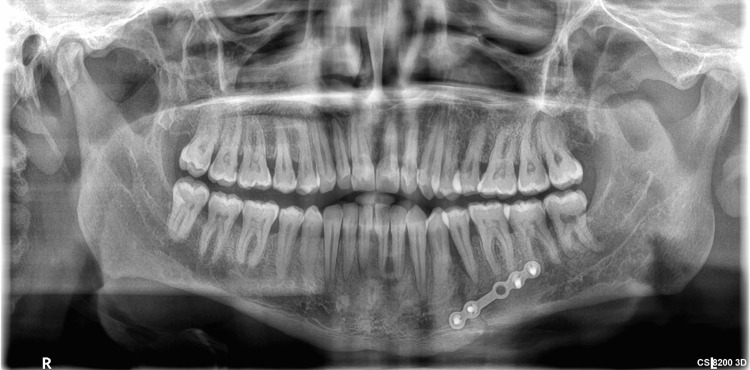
A postoperative orthopantomogram after four weeks, showing adequate bone healing.
